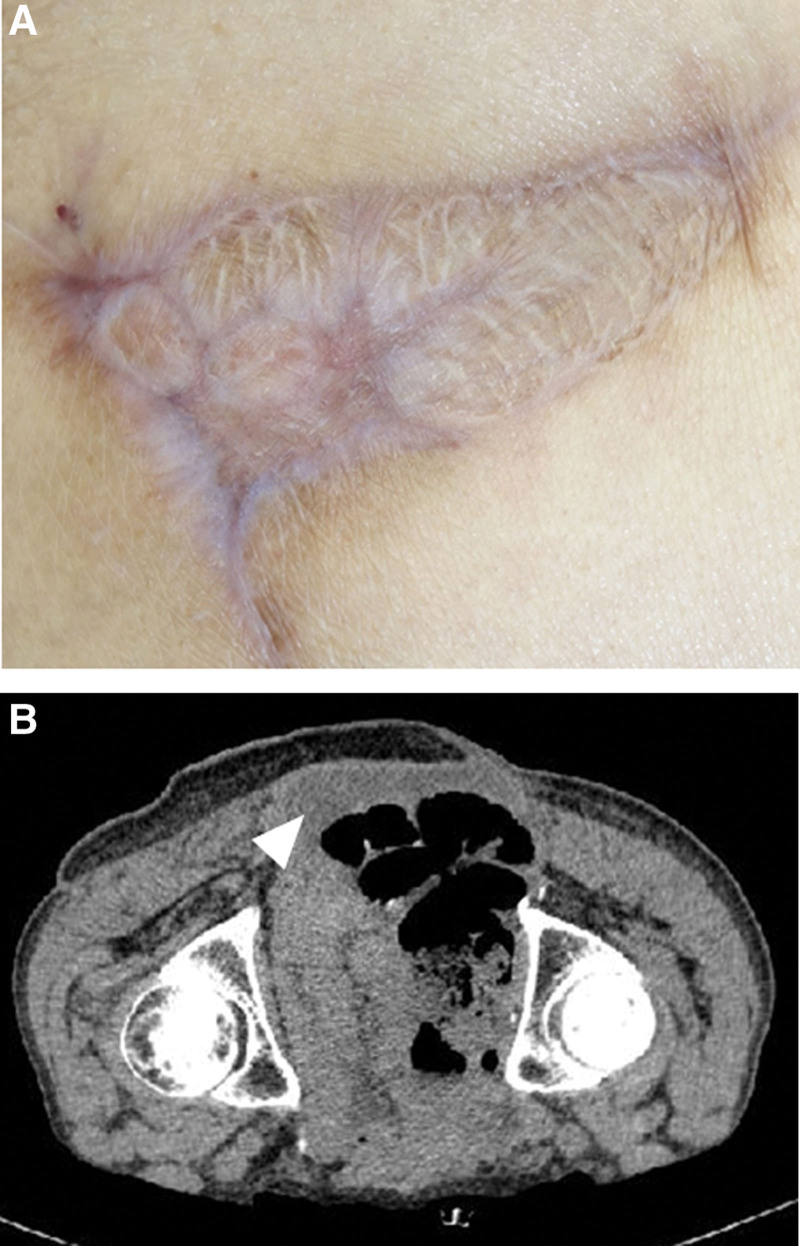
(a) Post flap surgery day 307. Secondary healing was complete; the skin ulcer did not recur; (b) Computed tomography image of wound site at post flap surgery day 307 with patient in the prone position. A small amount of fluid retention is observed between the retroperitoneum and flap (arrowhead). The retroperitoneum is not exposed to the external environment.
